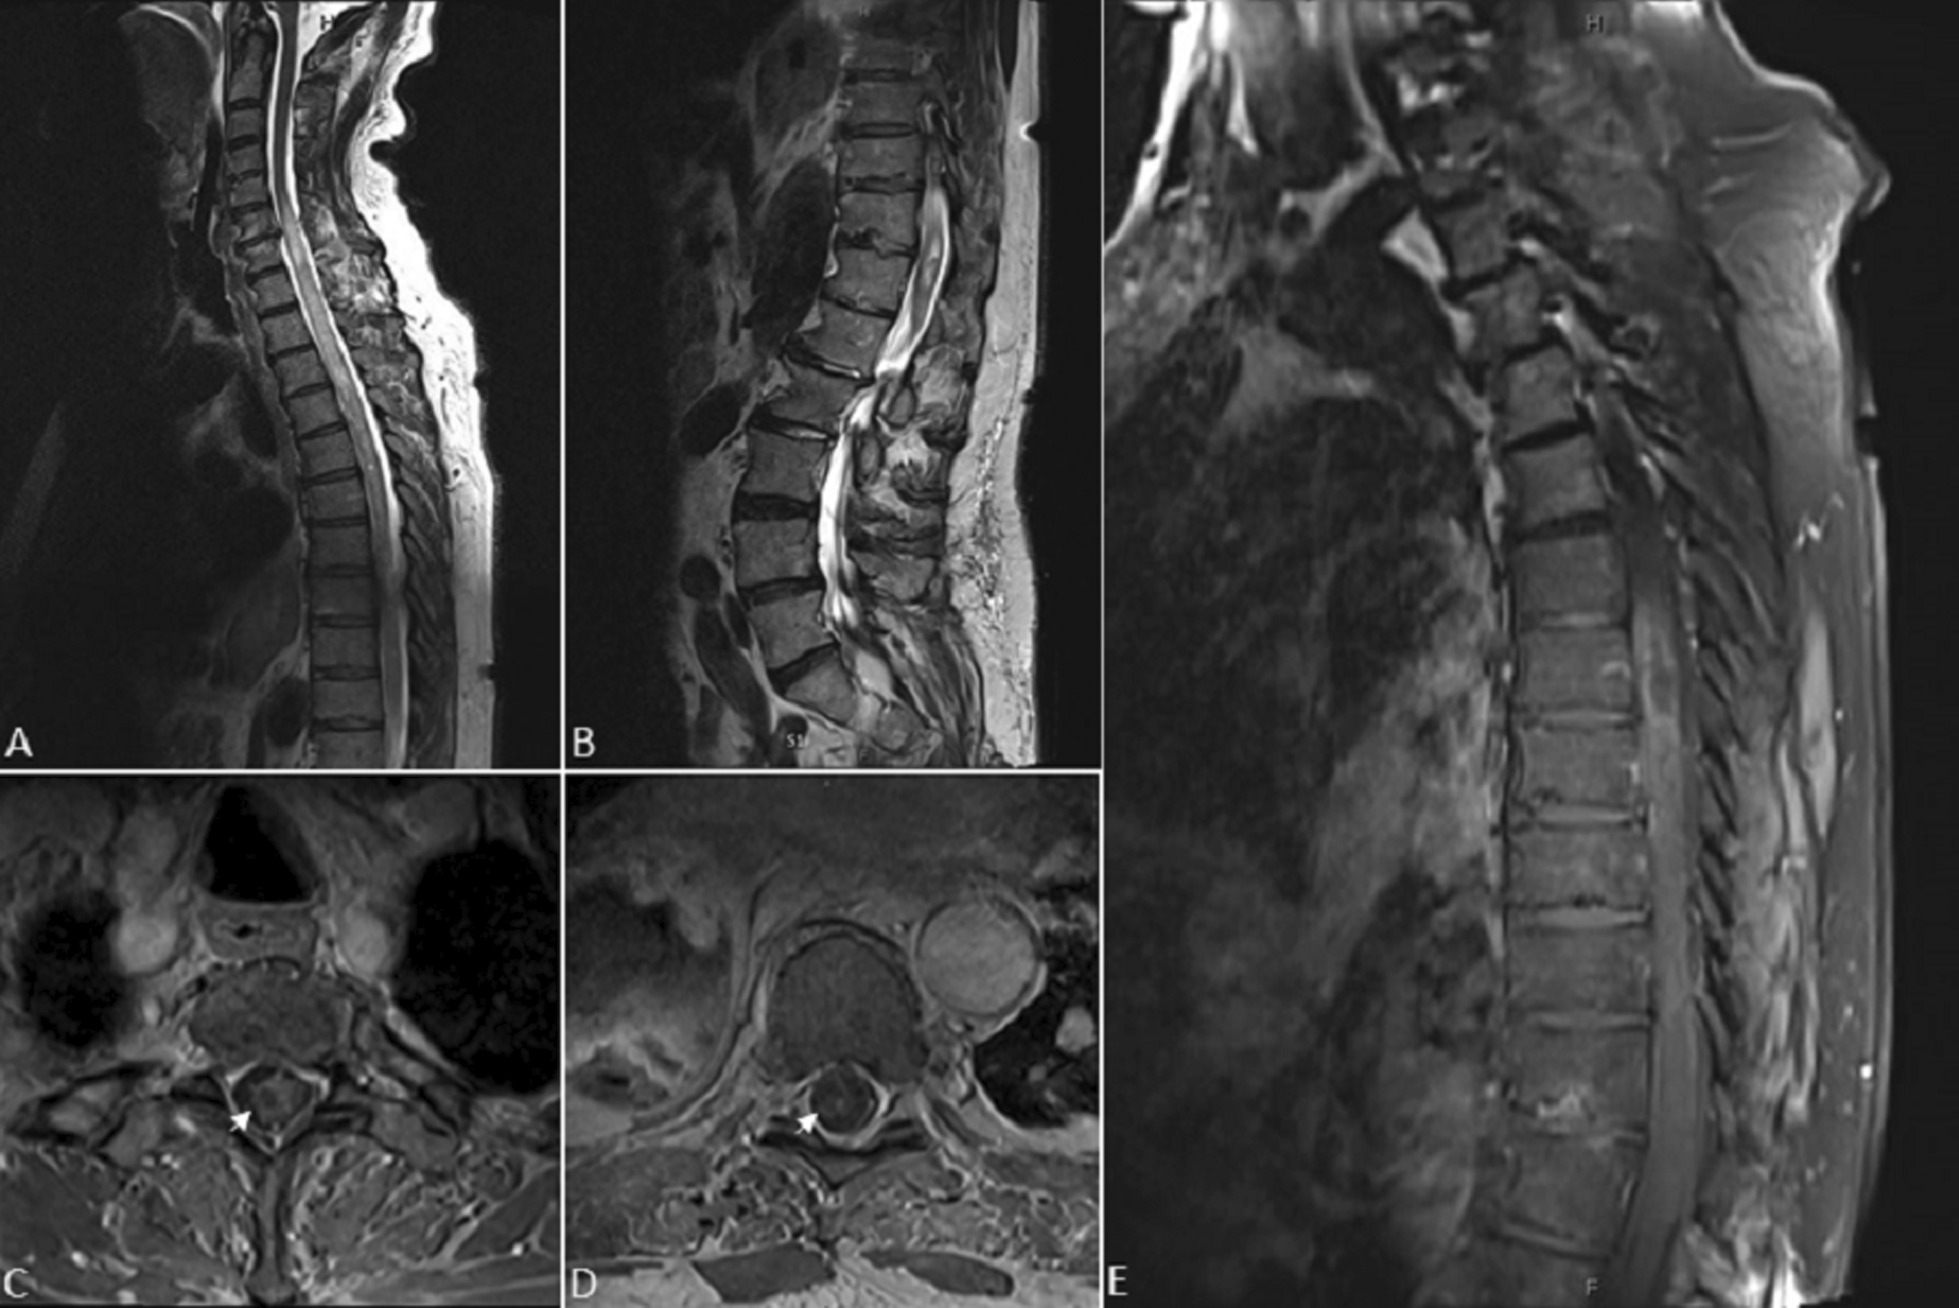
A Sagittal cervicothoracic and (B) lumbar spine T2-weighted MR images obtained several hours later with progression of expansile heterogeneous hyperintensity now extending from C5 to the conus medullaris. Axial T1 post-contrast images of the (C) upper and (D) lower thoracic spine demonstrate foci of ring enhancement within the right hemi-cord (arrows). E The peripheral ring-enhancement is also visible on sagittal T1 post-contrast images
